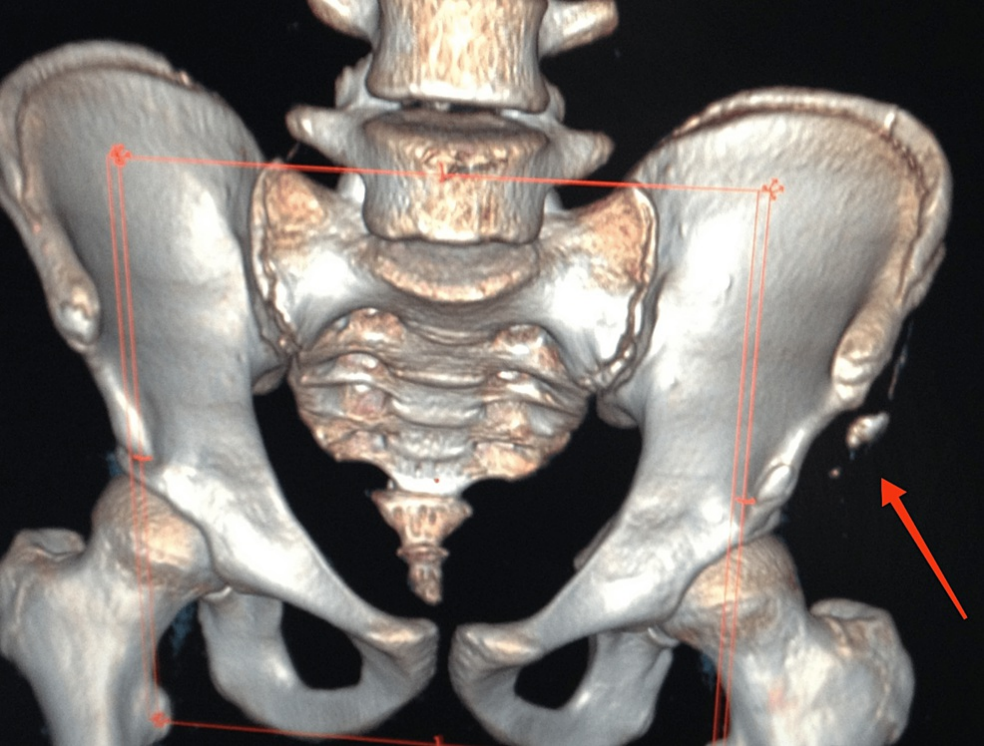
CT scan of the pelvis with 3D reconstruction 3D: Three-dimensional The red arrow is showing a fracture of the anterosuperior iliac spine.
